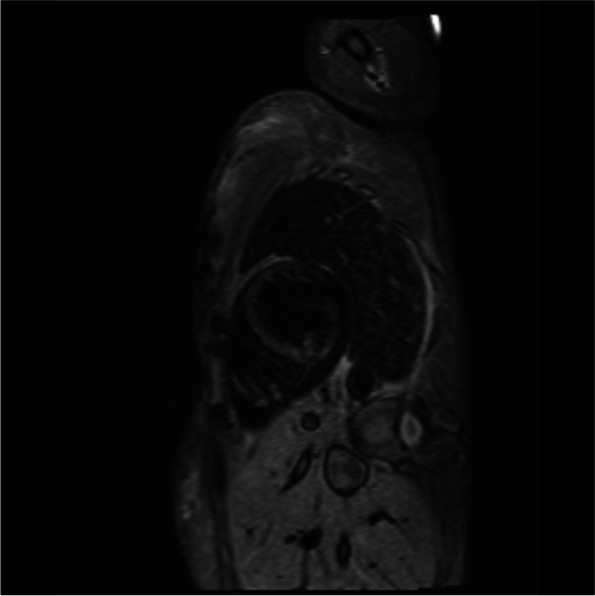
Cardiac MRI, T2-weighted stir sequence, midventricular short-axis view, showing an increased signal intensity of the mid inferior segment
